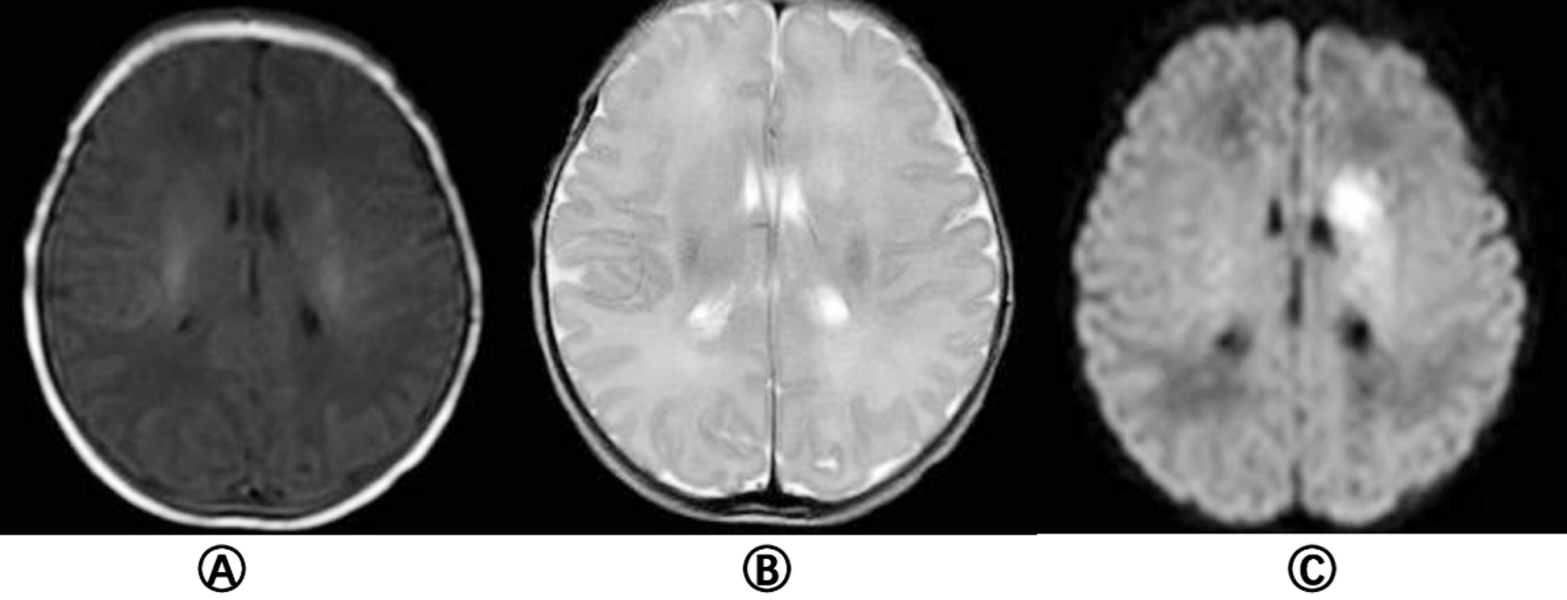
MRI findings of left basal ganglia in neonatal cerebral i nfarction a) T1WI showed long T1 signal in the left basal ganglia; B) T2WI showed long T2 signal in the lesion area; C) Diffusion weighted imaging (DWI) showed patchy hyperintensity in the left basal ganglia.
